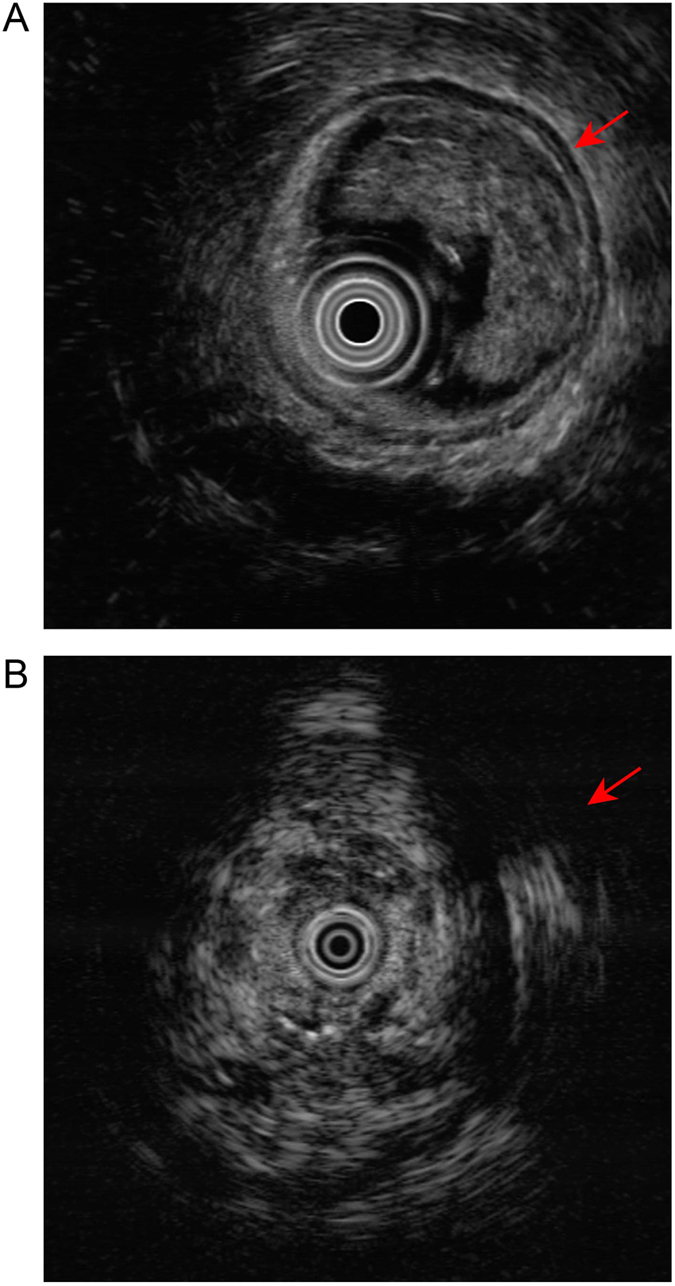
Endoscopic ultrasound images. (A) Pre-treatment; (B) Six months after radiotherapy.
